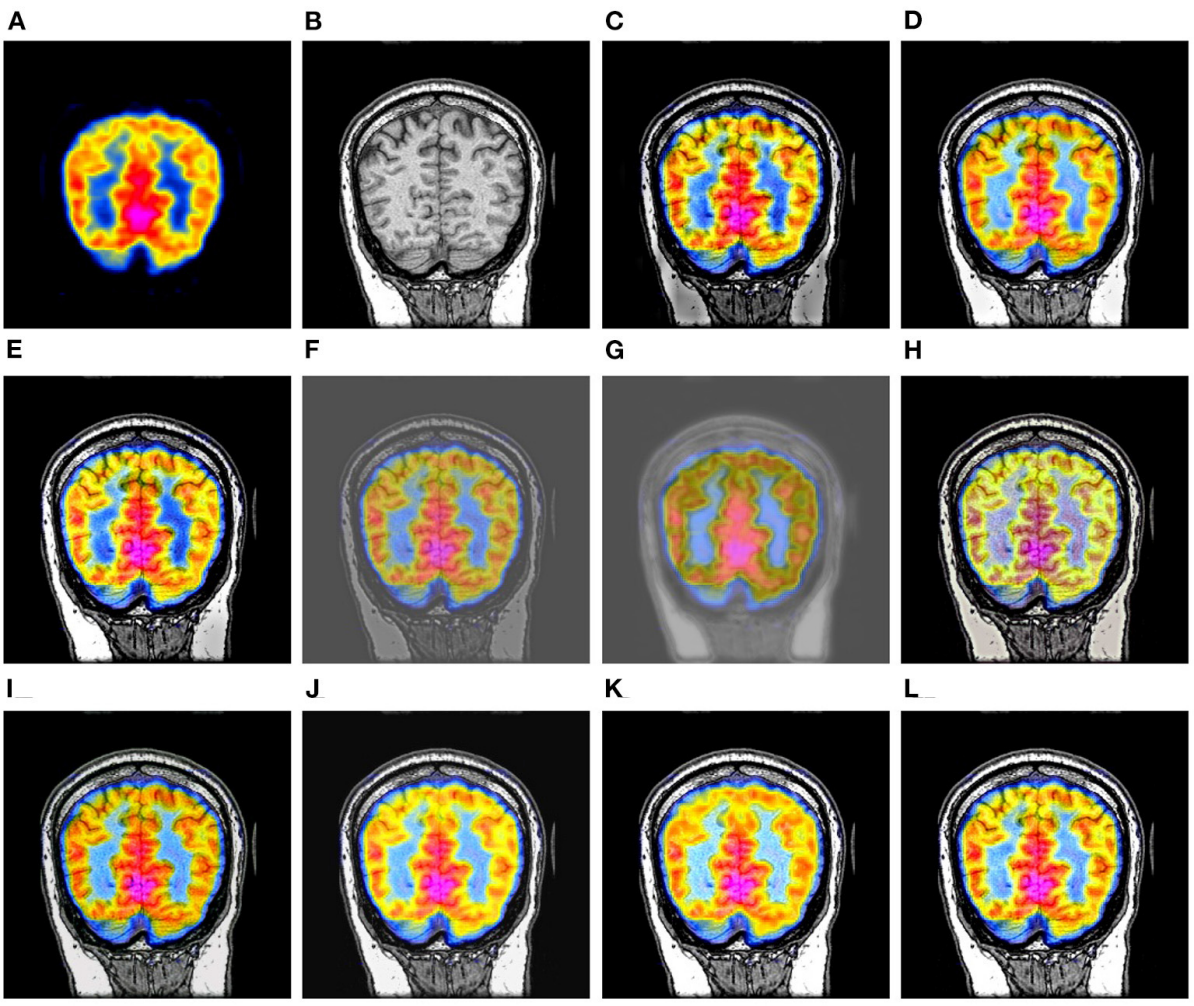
Comparison example on the PET-MR image dataset. (A,B) are the original PET image and MR image, respectively. (C–L) are the fusion results of DWT, GFF, LPSR, U2Fusion, DDcGAN, IFCNN, EMFusion, DRBIF, LEGFF_0_, and our method, respectively.
